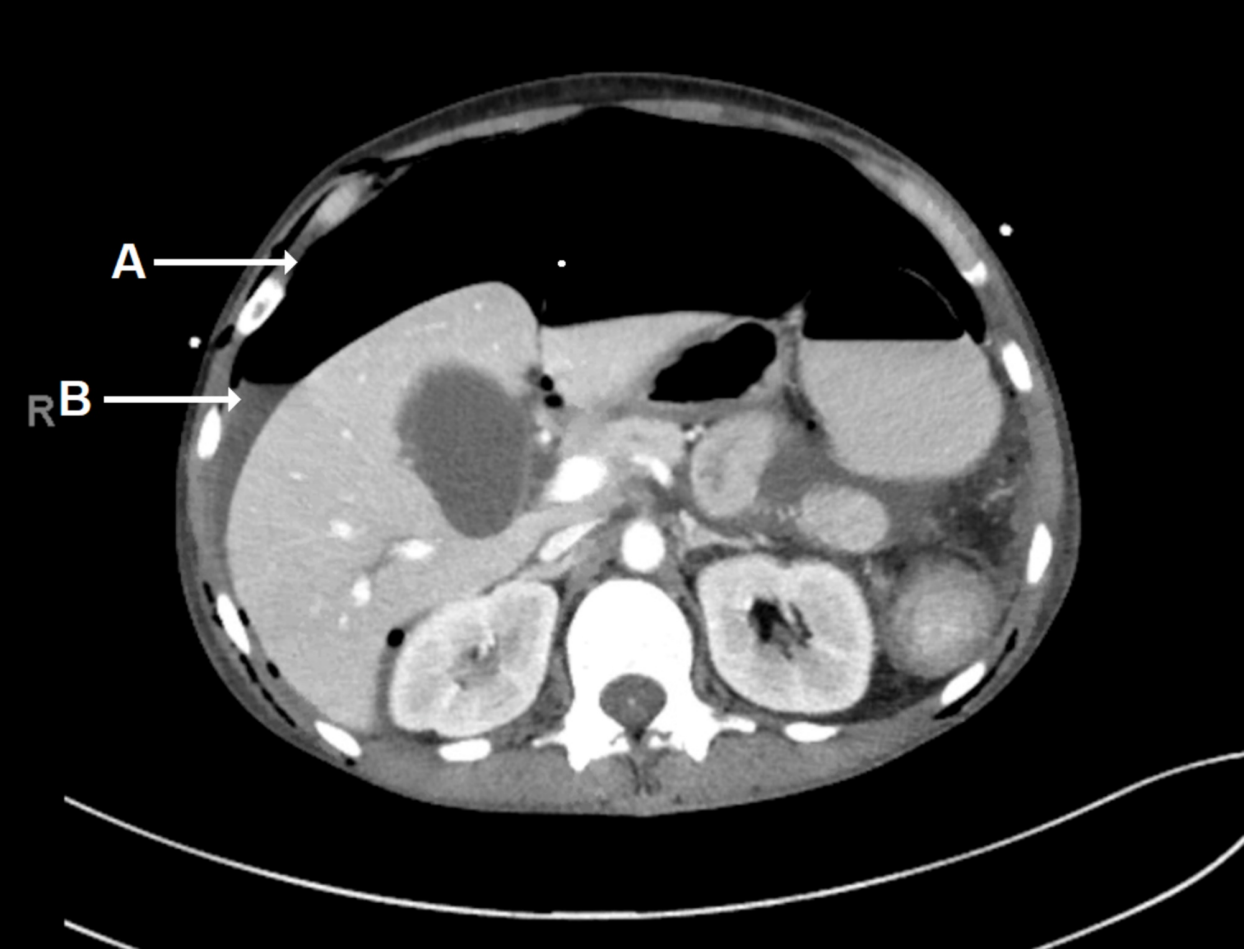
CT of the Abdomen/Pelvis CT of the abdomen/pelvis with intravenous contrast only, in the axial plane, demonstrating pneumoperitoneum (A) with small-to-moderate volume ascites (B).
